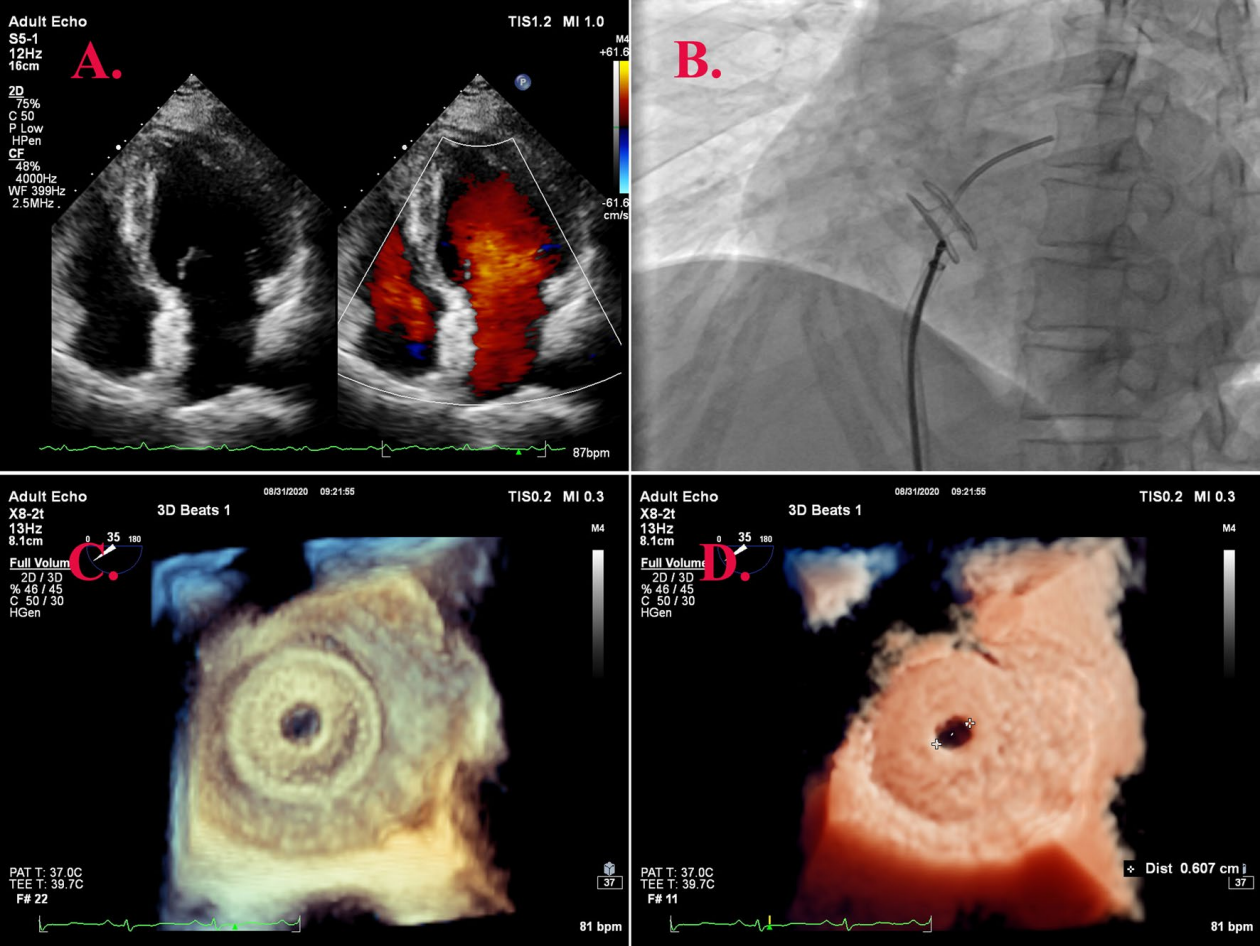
Color Doppler shows the shunt signal. A Echocardiogram showing D-shant atrial shunt discs tightly clamped on both sides of the atrial septum. Two-dimensional ultrasound findings are shown on the left and colour Doppler ultrasound findings are on the right. B Immediate left atrial pressure changes measured by catheterization after atrial shunt implantation in the atrial septum. C 3D transesophageal echocardiography (3D TEE) clear reconstruction of D-shant's left atrial disk slice. D The shunt orifice diameter of the D-shant was 0.6 cm measured under 3D TEE
